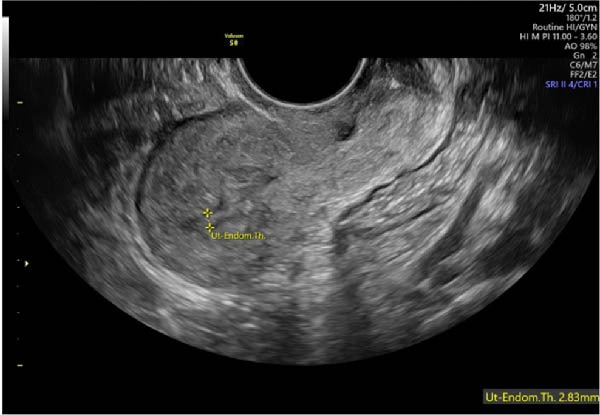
Transvaginal two‐dimensional gray scale ultrasonography of the uterus in the sagittal (longitudinal) plane.
